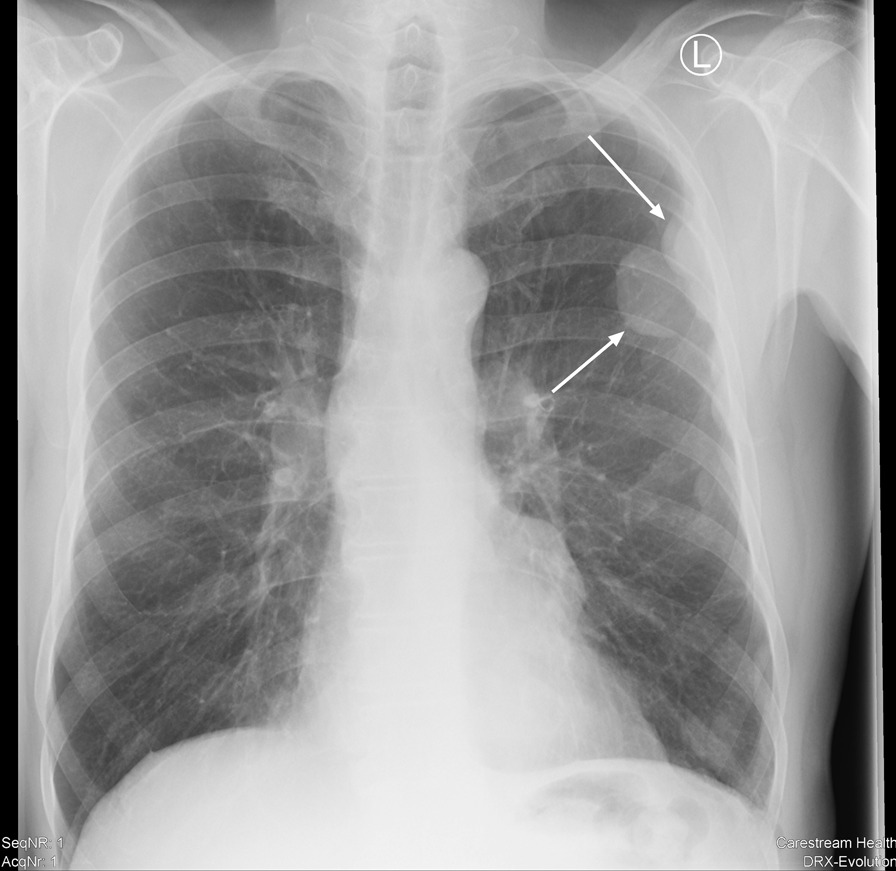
Chest X-ray at admission. White arrows indicate pleural masses, initially interpreted as possible mesothelioma
